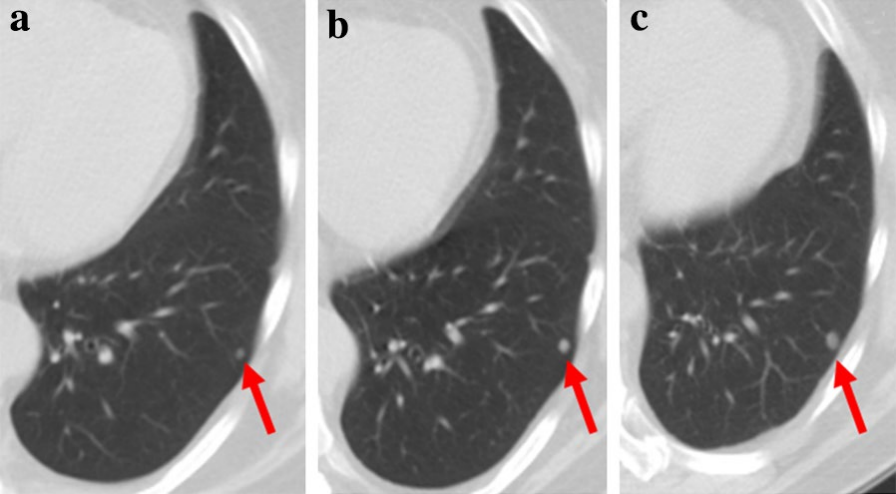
Axial CT images performed on 12/11/14 (a) and 1/23/15 (b) show interval growth of a *left lower lobe* nodule from 3 to 6 mm. Follow-up axial CT image performed on 2/1/16 (c) shows a grossly stable 6 mm *left lower lobe* nodule
